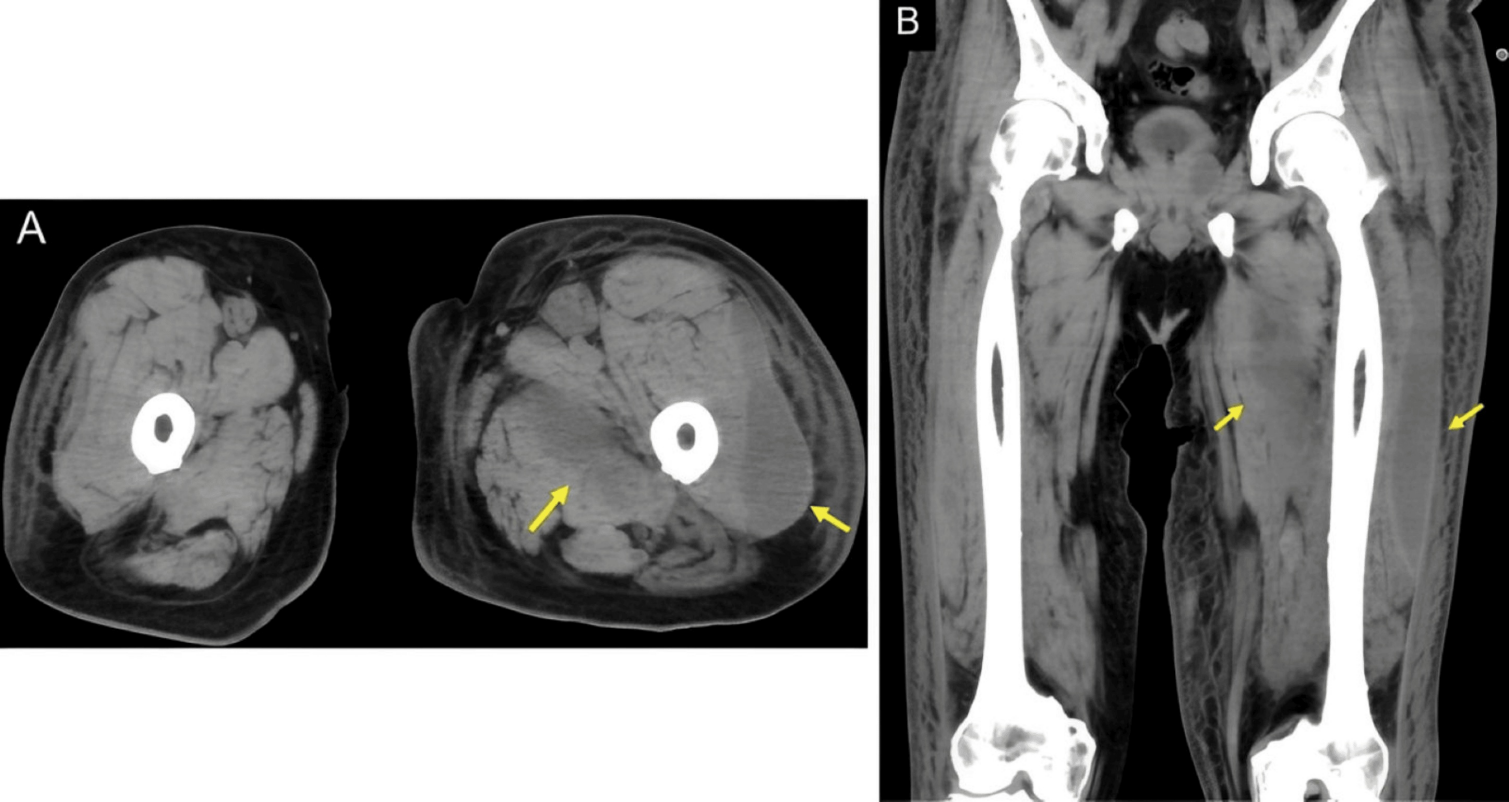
CT image of the thigh On day 17 after admission, axial (A) and coronal (B) CT images reveal a swollen left thigh with intramuscular fluid retention within the vastus lateralis (arrow) and adductor brevis muscles.
